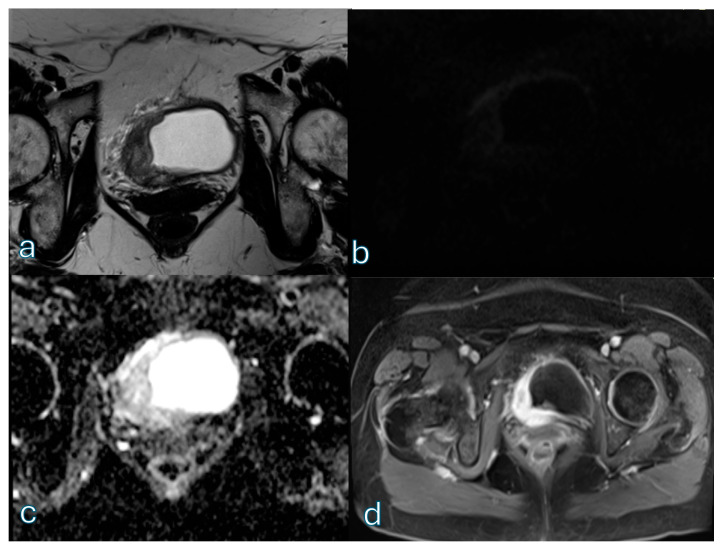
T2w Multiparametric MRI (a) shows a lesion arising from the right lateral bladder wall with morphologic features suspicious for high-risk disease. On diffusion-weighted imaging (DWI, (b)), the lesion does not demonstrate significant diffusion restriction, with no corresponding low signal on the ADC map (c ), a finding that could potentially lead to underestimation or misclassification. In contrast, dynamic contrast-enhanced (d) imaging demonstrates early enhancement with interruption of the muscularis propria without extravesical extension, allowing accurate classification as VI-RADS 4.
